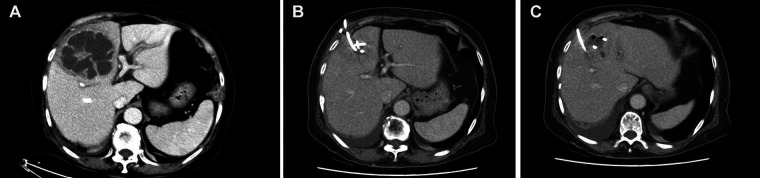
(A–C) two pigtail drainage catheters in the PLA and significant reduction of pyogenic liver abscess was observed on postoperative abdominal CT.
